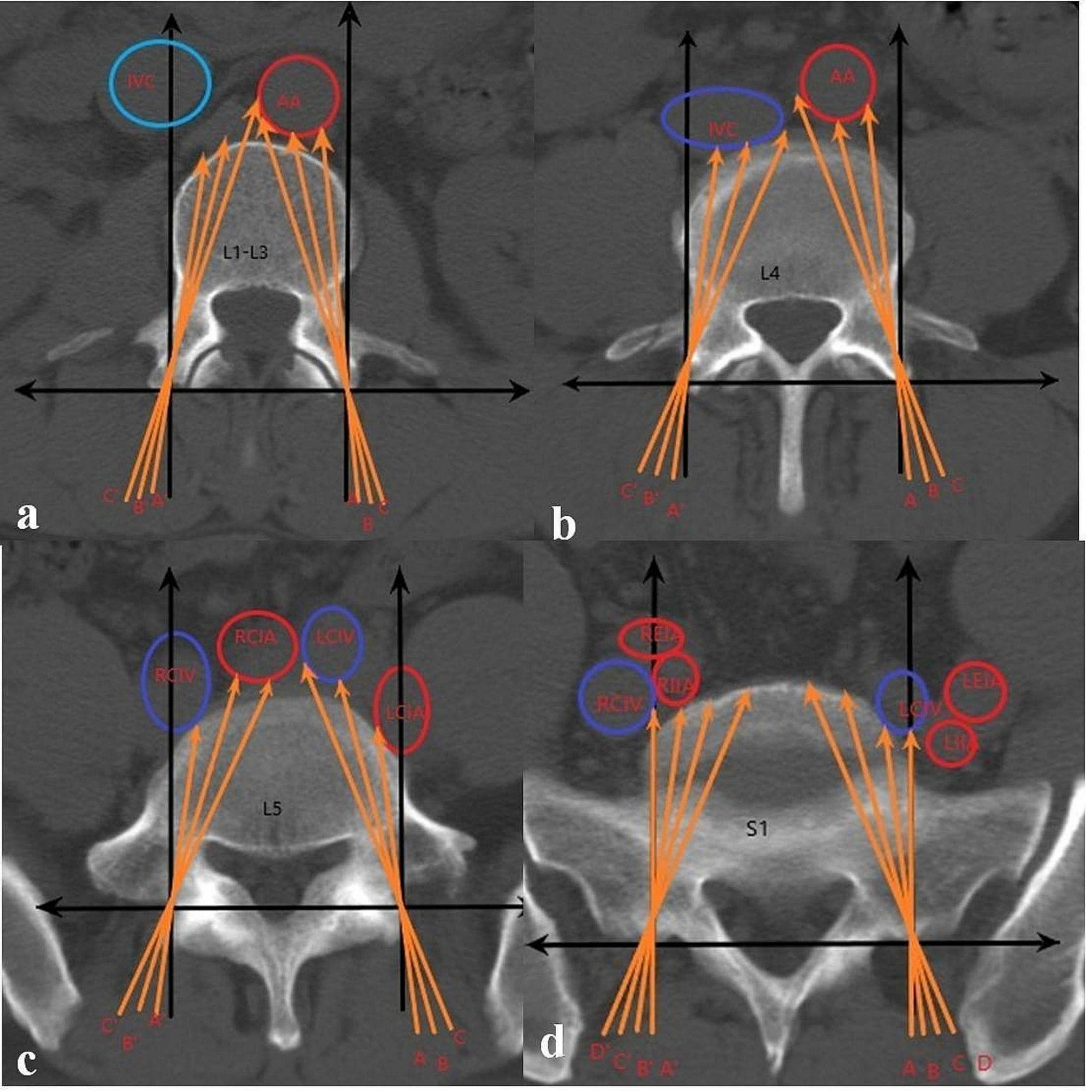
The distance from the needle insertion point to the adjacent blood vessels of the vertebral body from CT images. AA: abdominal aorta, IVC: inferior vena cava, LCIA: left common iliac artery, RCIA: right common iliac artery, LCIV: left common iliac vein, RCIV: right common iliac vein. LEIA: left external iliac artery, LIIA: left internal iliac artery, REIA: right external iliac artery, RIIA: right internal iliac artery, INTA: introversion angles, L: lumbar, S: sacral. At L1-L5 (figure a, b, c), A, B and C represent the left INTA with 5°, 10° and 15°, respectively; A′, B′ and C′ represent the right INTA with 5°, 10° and 15° respectively. At S1 (figure d), A, B, C ,D represent the left INTA at 0°, 5°, 10° and 15°, respectively; A′, B′, C′ and D’ represent the right INTA at 0°, 5°, 10° and 15° respectively
